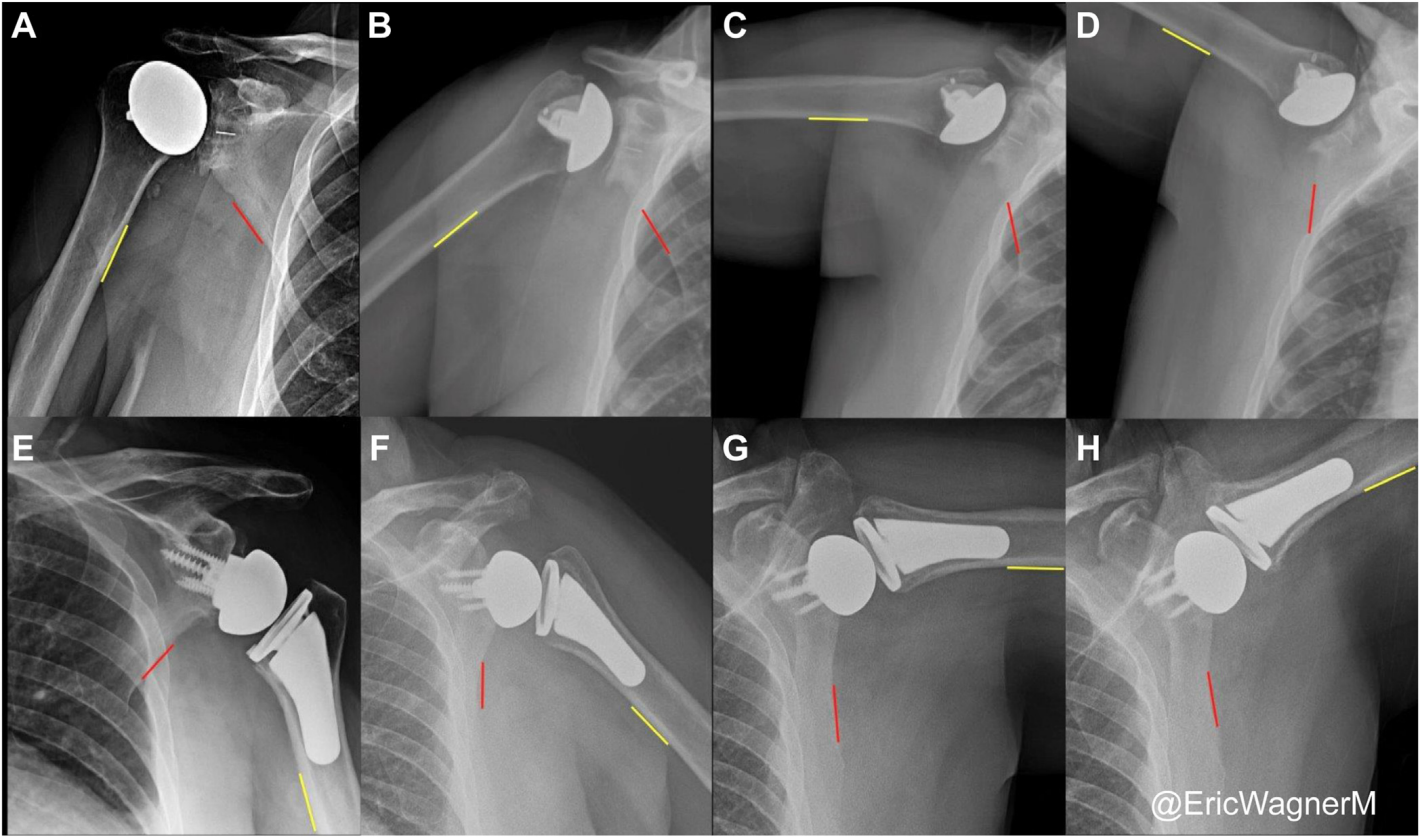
Radiographs showing glenohumeral and scapulothoracic abduction in a patient with aTSA (*top row*) and rTSA (*bottom row*) at rest (A and E), 45° abduction (B and F), 90° abduction (C and G), and 120° abduction (D and H). *aTSA*, anatomic total shoulder arthroplasty; *rTSA*, reverse total shoulder arthroplasty. *Red line* represents lateral border of the scapula, *yellow line* represents medial border of humerus.
